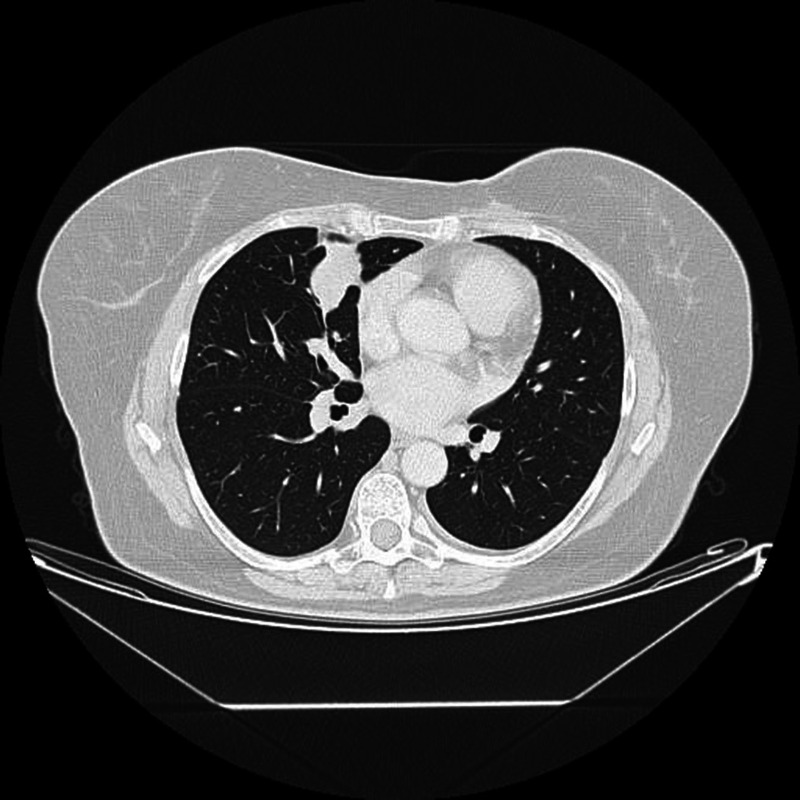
The timeline of the diagnostic tests and treatments. BI-RADS – breast imaging-reporting and data system; MDT – multidisciplinary team; uLMS – uterine leiomyosarcoma; CT – computed tomography.
